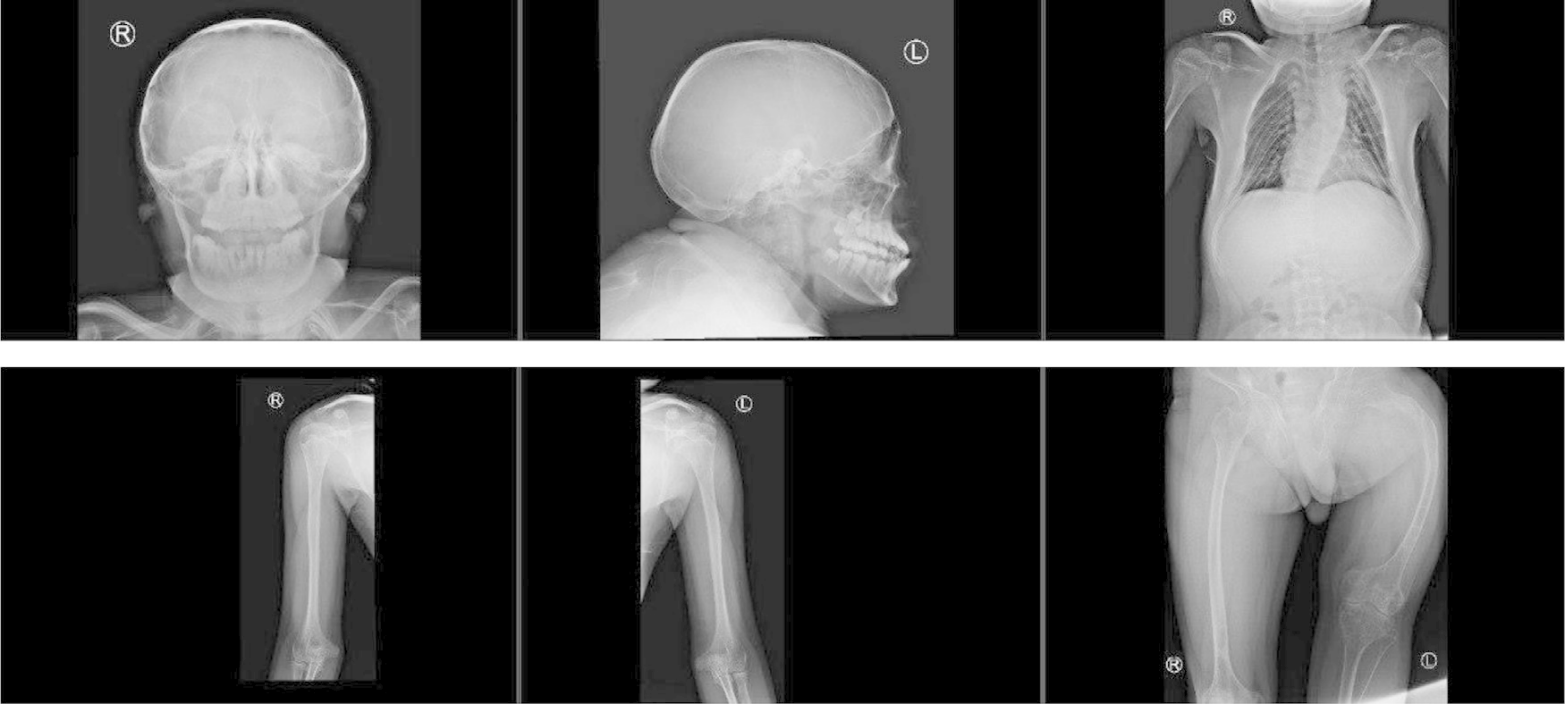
X-ray of the patient shows decreased bone density, deformities of the thoracolumbosacral vertebrae with a codfish appearance and platyspondyly, malunion of a fracture in the proximal third of the left femur, and bilateral femoral bowing, more pronounced on the left.
